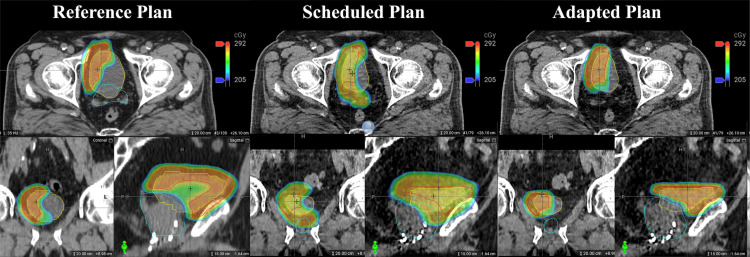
Anatomic comparison of dose distribution in Case 2 For Case 2, sample dosimetry for the reference plan on the planning CT anatomy and scheduled and adapted plans based on the CBCT and anatomy of the day. The lowest portion of the dose colorwash is the Dmax, 0.03 cc goal for the avoidance region (cyan). For each, the top, bottom left, and bottom right sub-images are axial, coronal, and sagittal views, respectively. At treatment, the bladder fill is appreciably less, resulting in normal tissue overdose and target under coverage without plan adaptation.
